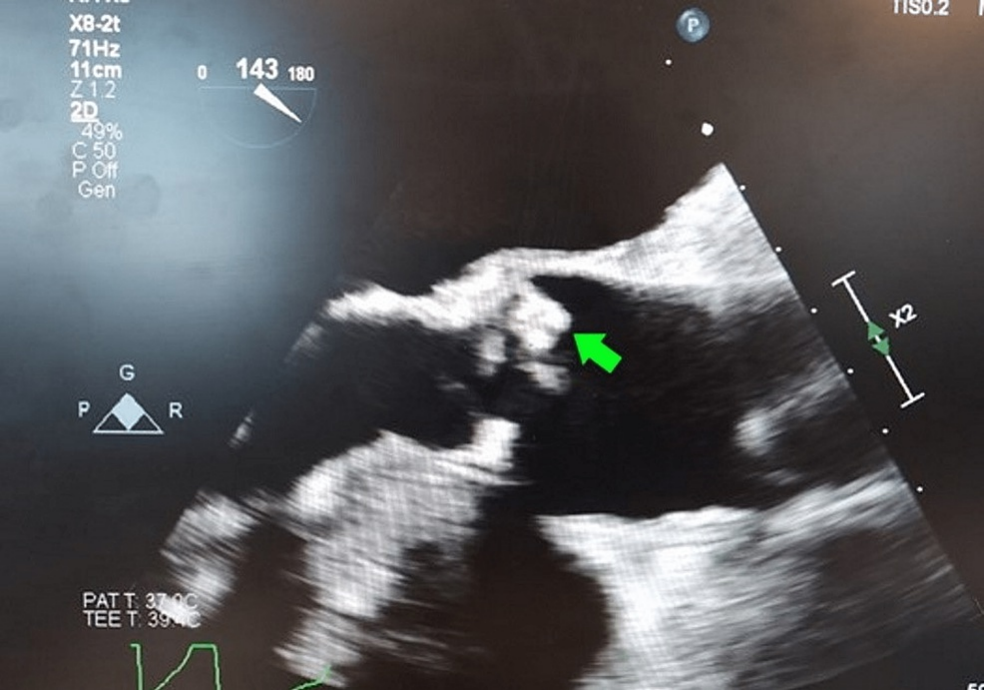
Transesophageal echocardiogram showing an aortic mass
